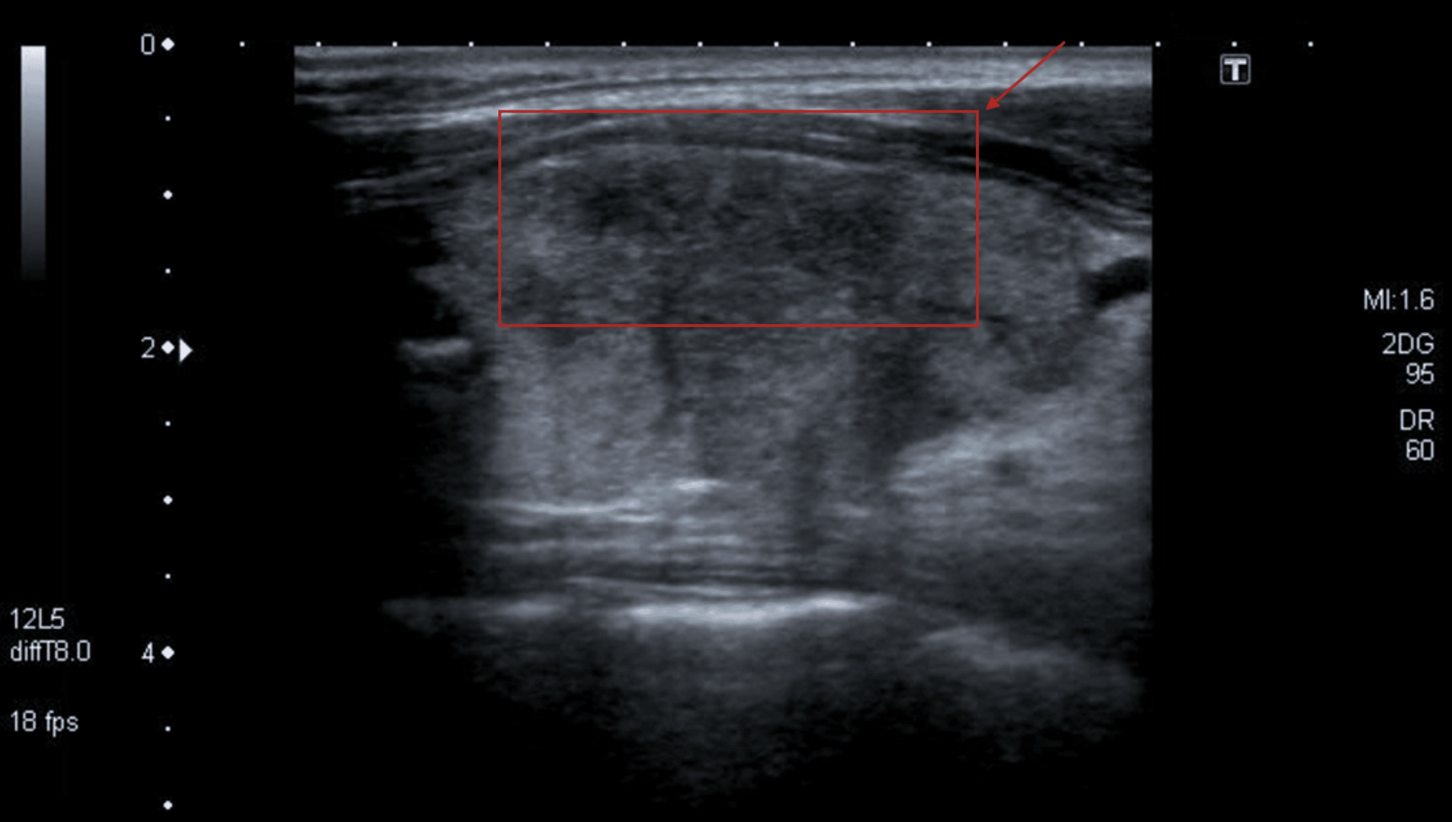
Ultrasound findings in subacute thyroiditis: confluent, hypoechoic region in thyroid parenchyma
